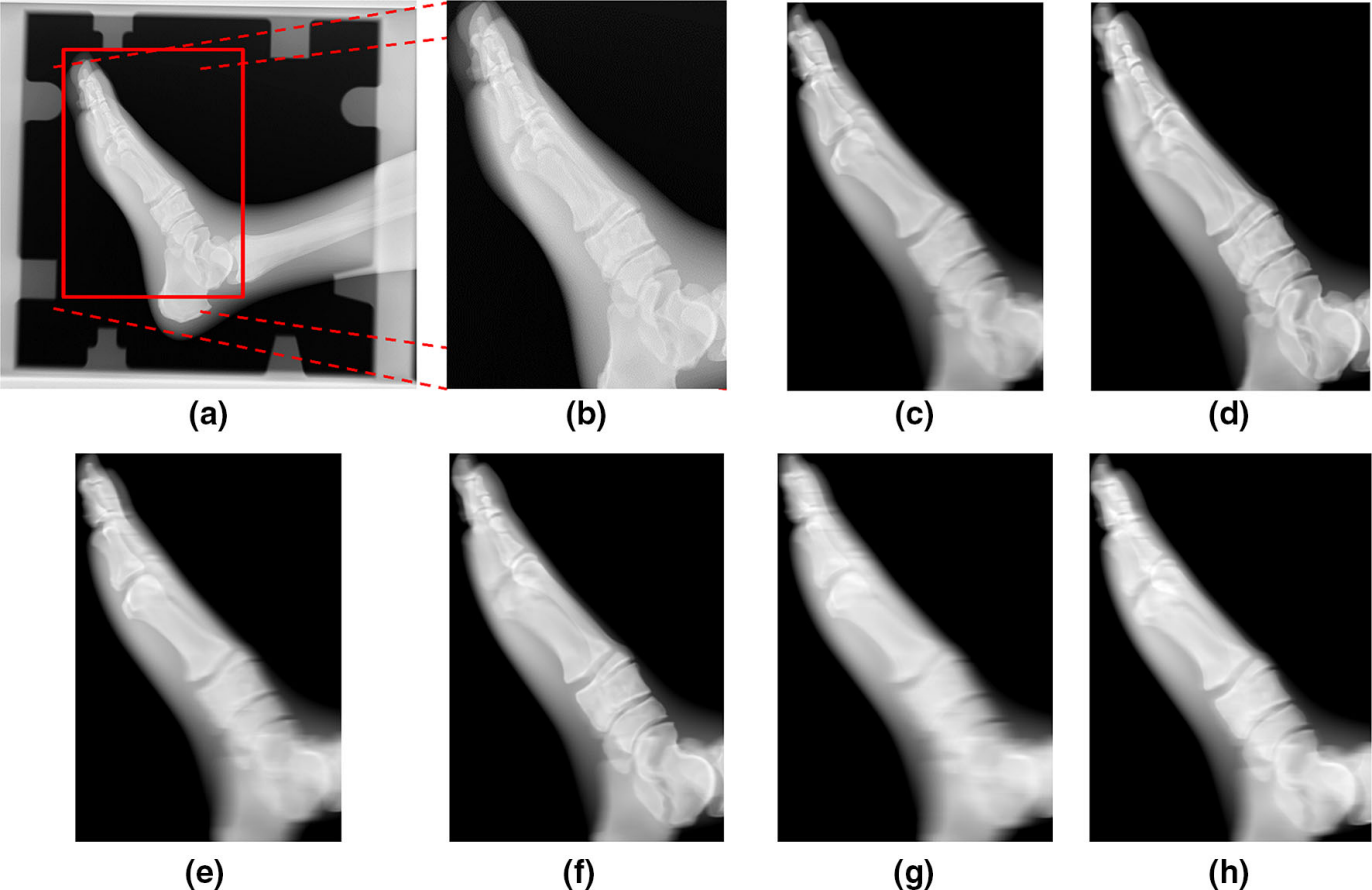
X-ray image and reconstructed tomosynthesis. a Original X-ray image. b Enlarged image around the *red rectangle* in (a). c, d Tomosynthesis images obtained by changing the depth of interest (line trajectory). e, f Tomosynthesis images by changing the depth of interest (circular trajectory). g, h Tomosynthesis images by changing the depth of interest (complex trajectory)
